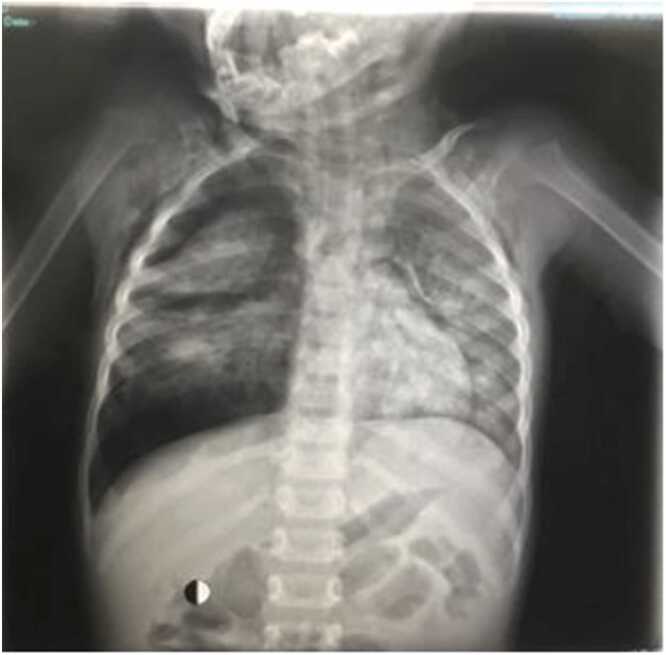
Chest radiograph demonstrating subcutaneous emphysema and pneumomediastinum in a 12-month-old male patient with measles-associated severe community-acquired pneumonia (Case 3).
